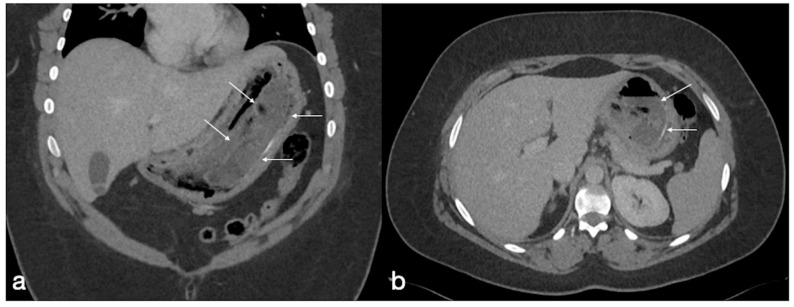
Deflated gastric balloon. A 17-year-old female patient with gastric balloon placed two months before, came to the emergency department complaining of a change in urine color due to spreading of methylene blue from the balloon lumen. A CT was requested to evaluate balloon position. The deflated balloon is still in the gastric cavity (a, coronal oblique view, b axial view, arrows).
